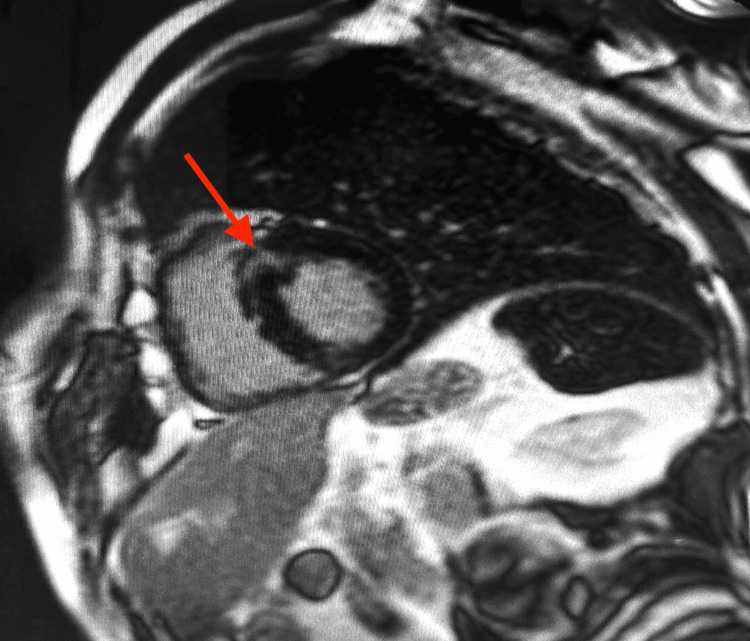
Cardiac Magnetic Resonance Late Gadolinium Enhancement of the Basal Septum PSIR late gadolinium enhancement cardiac magnetic resonance image in the basal short-axis view demonstrating focal hyperenhancement in the basal septum (red arrow), consistent with myocardial fibrosis.
